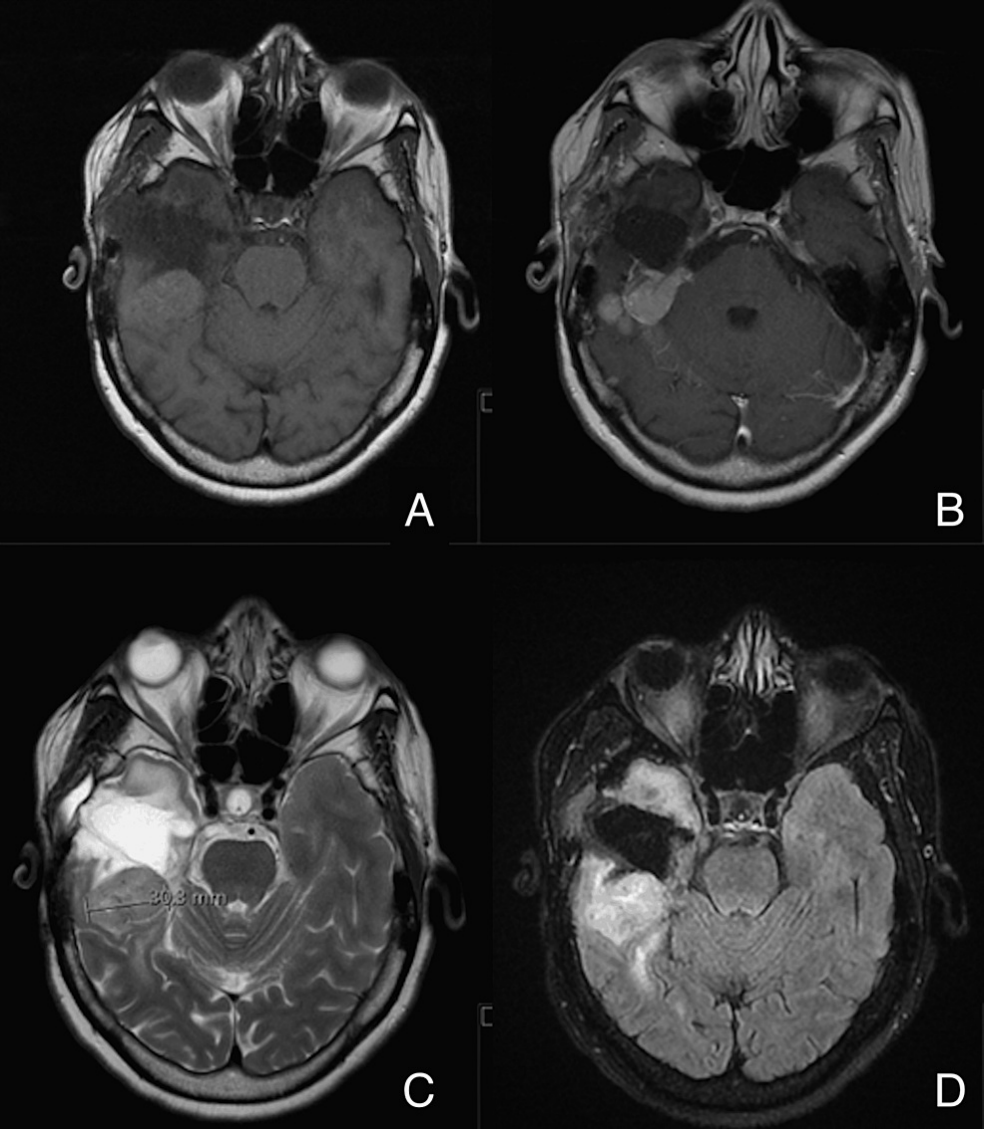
Petrous apex hemangiopericytoma magnetic resonance imaging (MRI) A: T1; B: T1 + contrast; C: T2; D: FLAIR imaging. FLAIR: Fluid-attenuated inversion recovery.
